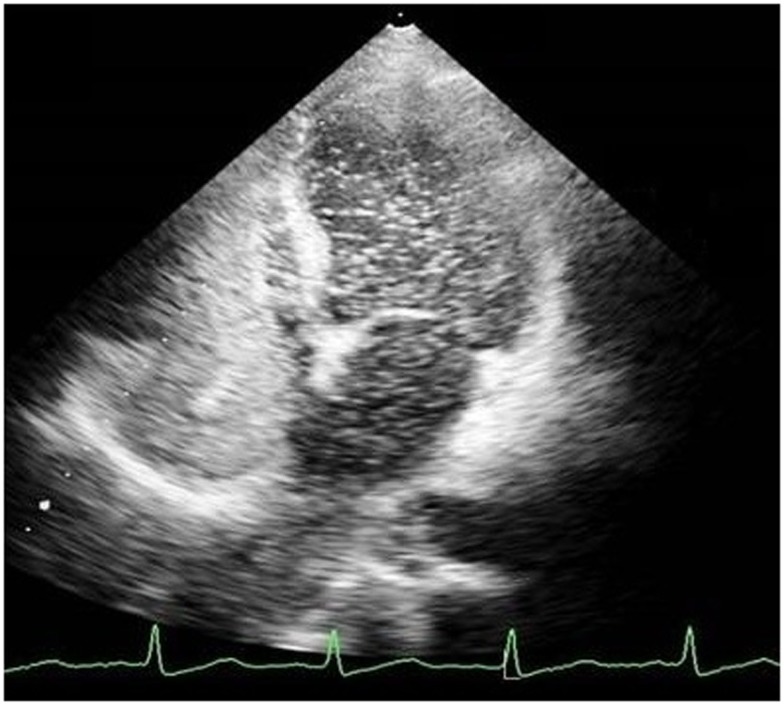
A pulmonary AVM as seen on echocardiogram.
